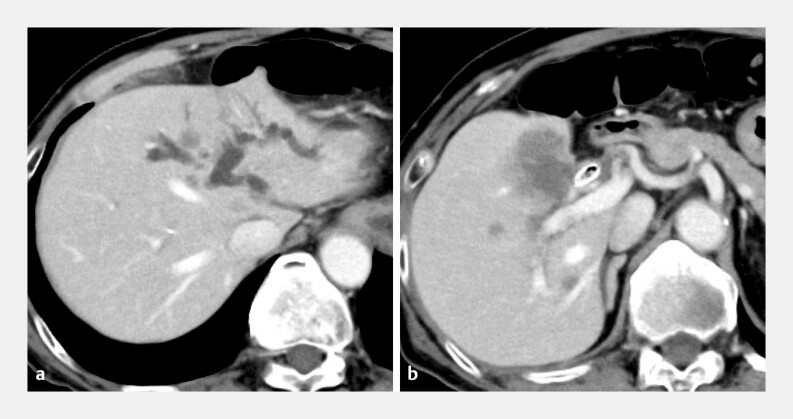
Computed tomography findings before endoscopic ultrasonography-guided hepaticogastrostomy.
